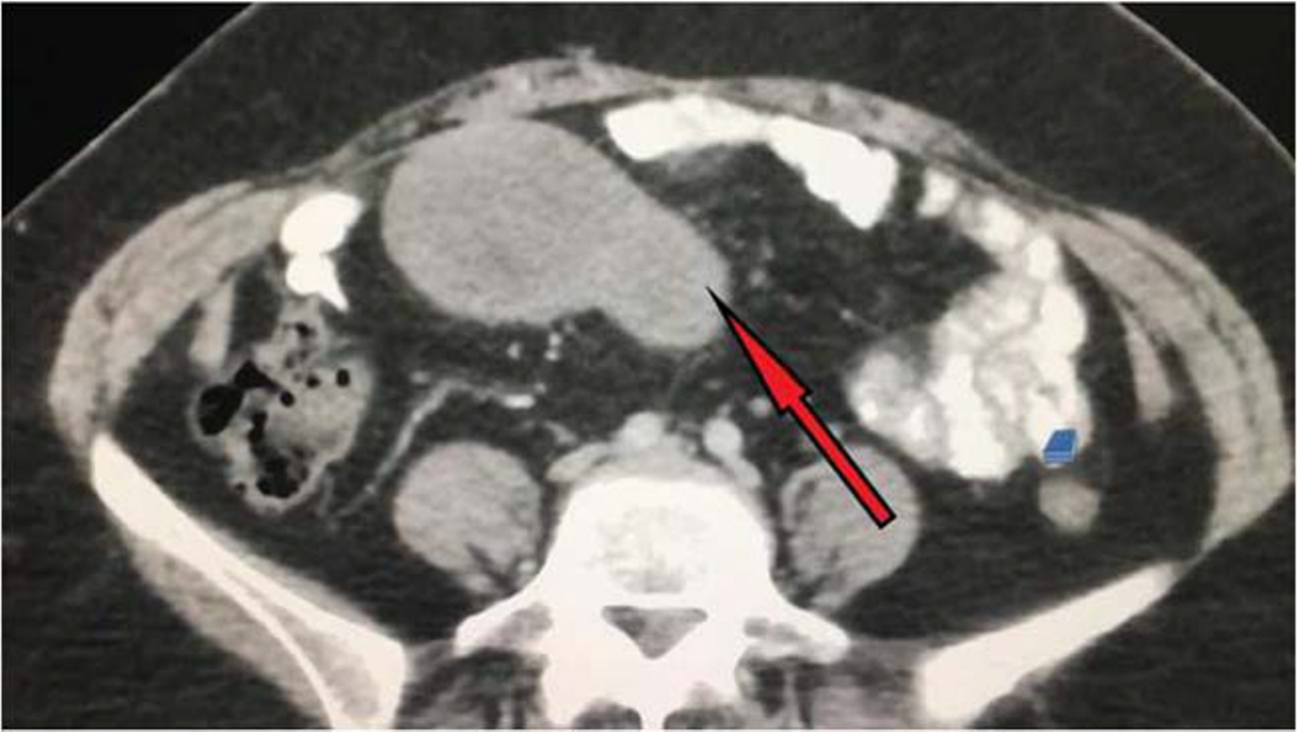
CT scan sagittal section image shows a dumbbell-shaped tumor (marked by red arrow) attached to the small intestine
